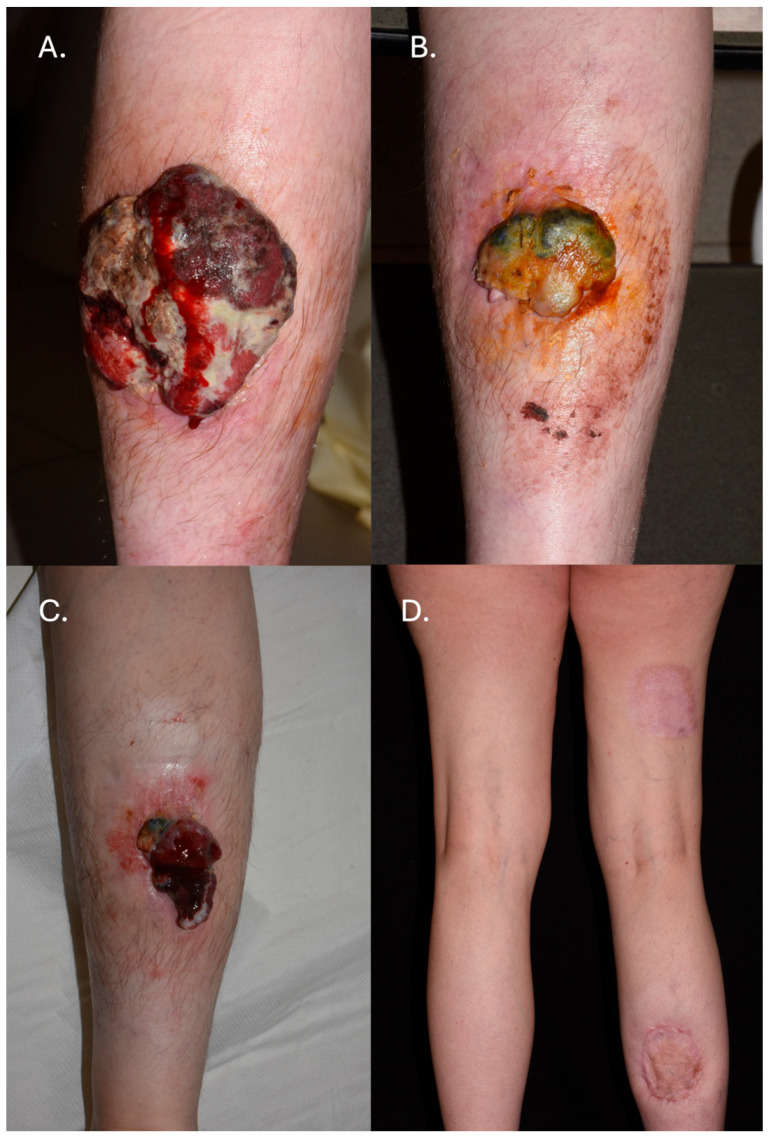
The primary melanoma at the time of the patient’s initial presentation in December 2019 (A); regression of primary melanoma at 6 months of dabrafenib and trametinib treatment (June 2020). At this time, pulmonary metastases were detected on CT scan (B); tumor size 8 months after starting ipilimumab and nivolumab therapy (C); after surgical removal of the tumor (D).
